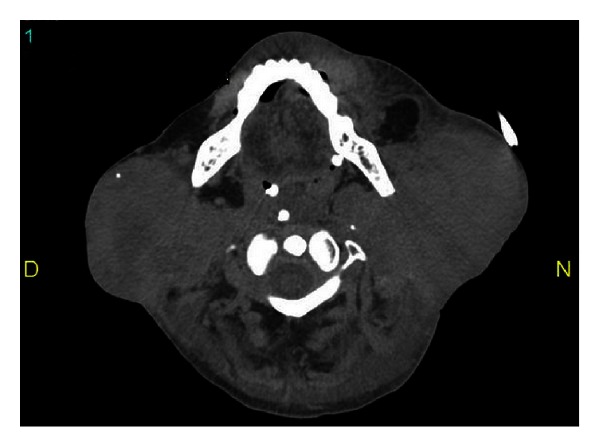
Neck computed tomography shows lymph node clusters in the neck associated with bilateral increase in size of parotid tissue.
